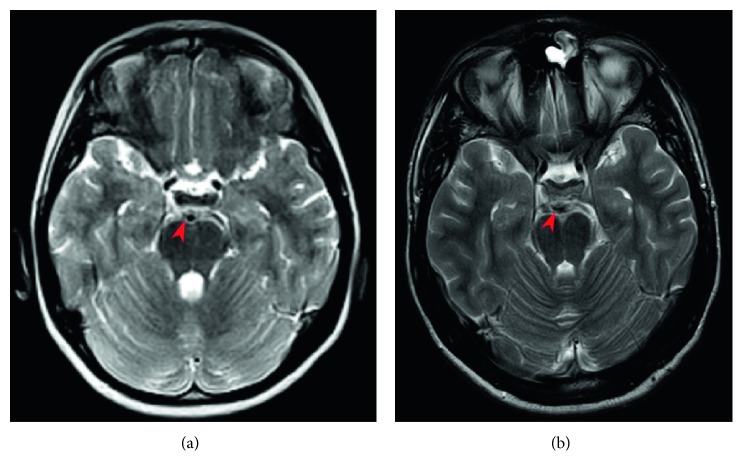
Axial T2-weighted MRI images comparison: (a) no focal or wide thickening of the oculomotor nerve during an acute attack of OM (Jan 2011); (b) 5-6 mm nodular mass suggesting an oculomotor nerve schwannoma (Oct 2018).
